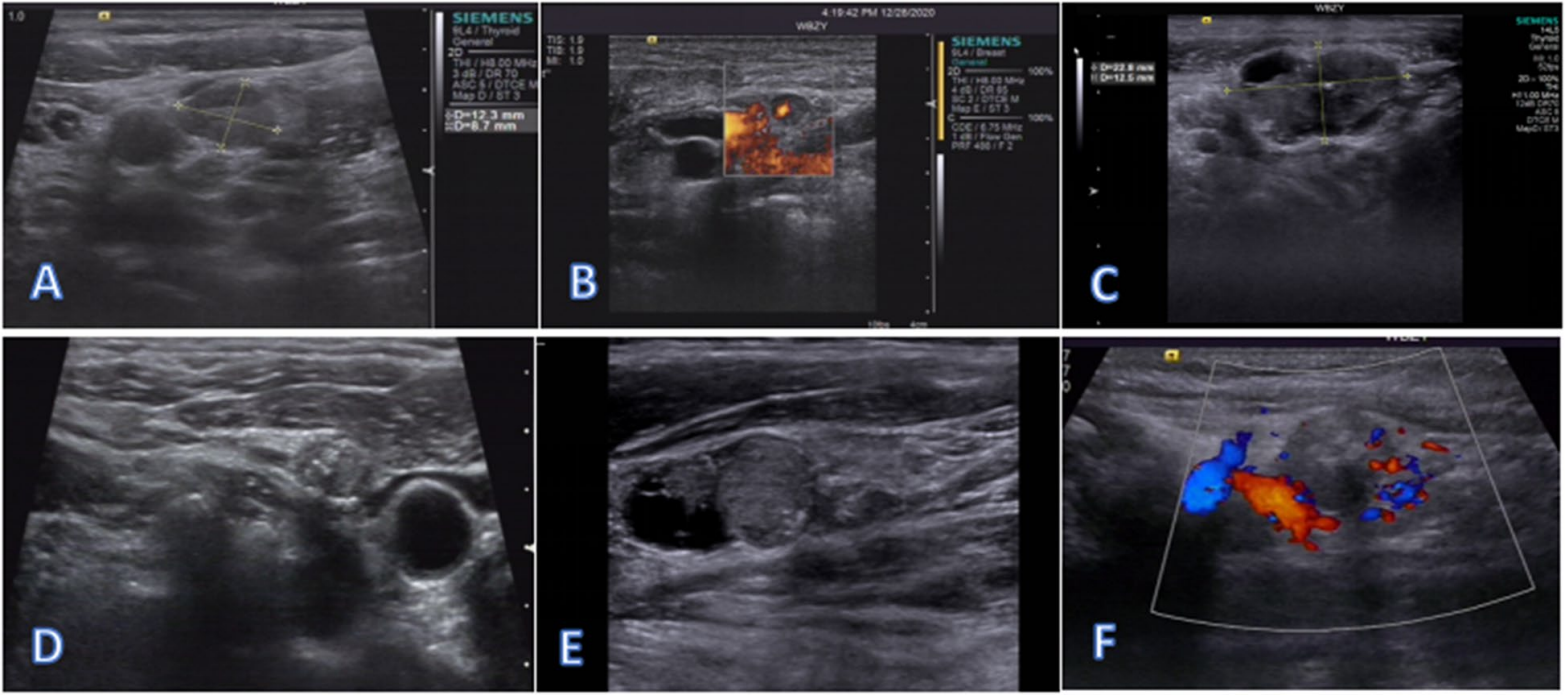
Different ultrasonic pictures of lymph node metastasis. A Cortical thickening. B aspect ratio > 1. C The lymphatic hilum was blurred or had disappeared due to cortical thickening. D Uneven Echo. E Cystic degeneration. F Increased blood flow signals
